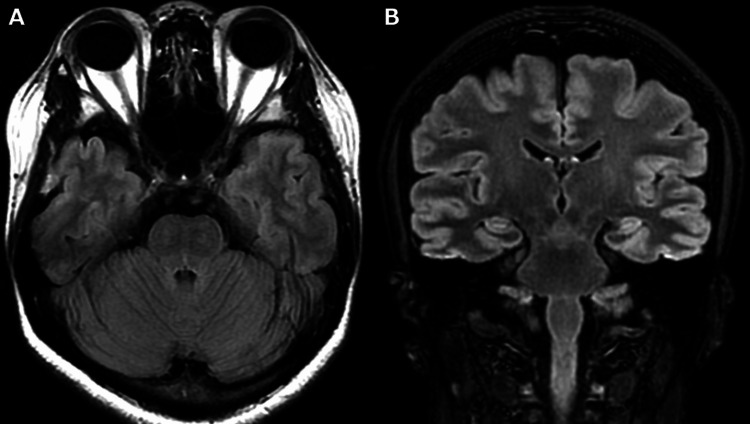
MRI on day 164. Fluid-attenuated inversion recovery (FLAIR) imaging following acute treatment indicates resolution of the lesion (A and B).
